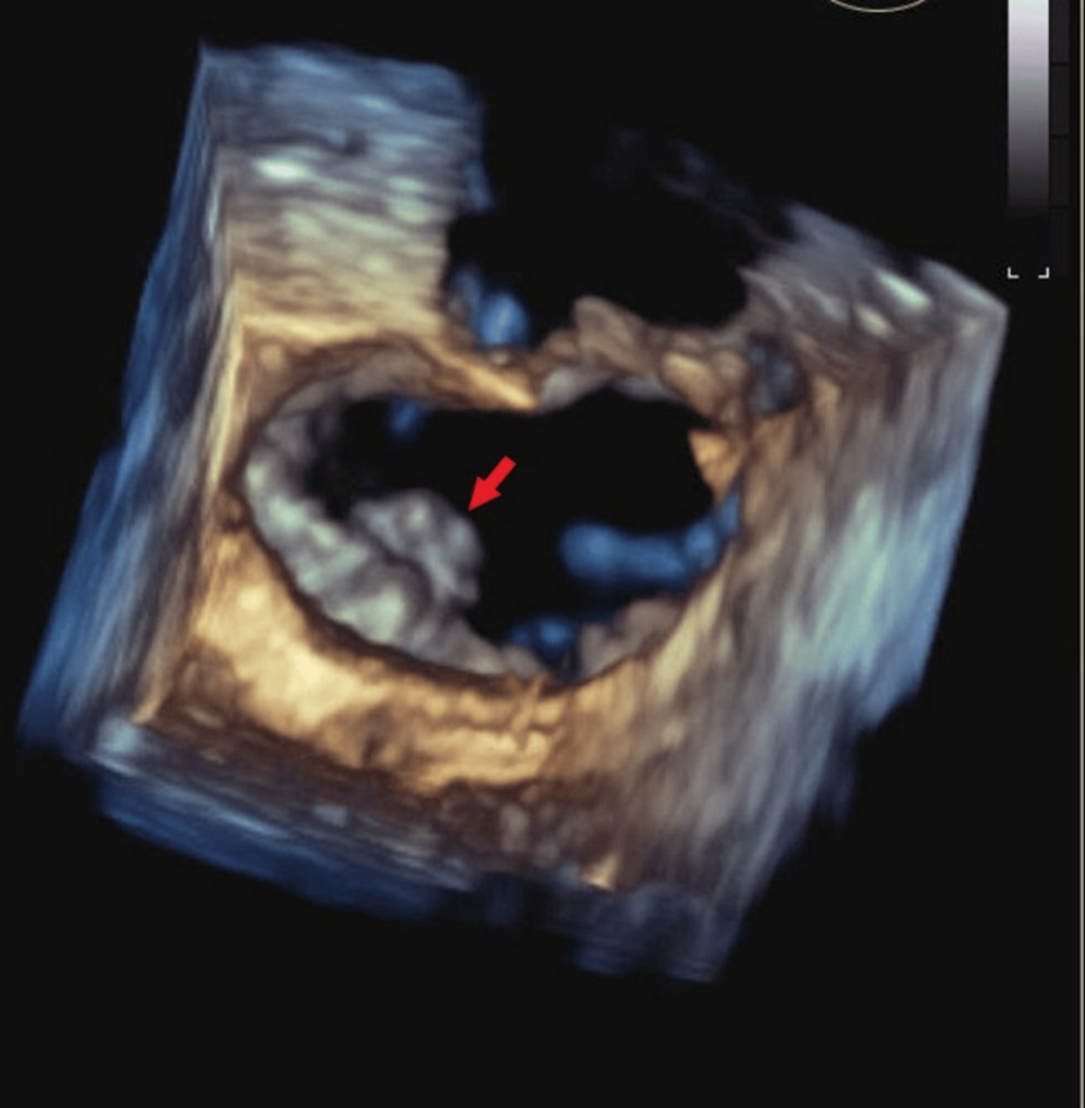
Transesophageal echocardiography This image demonstrates a mass on the atrial side of the posterior mitral valve leaflet (P2), measuring 8 × 5 mm (arrow) and attached to the base of the leaflet. No significant mitral regurgitation is associated with this finding.
